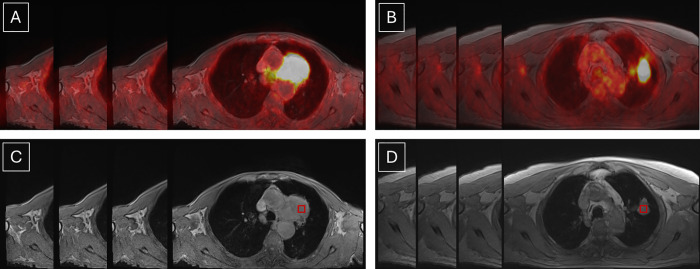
Images and selected regions of interest (ROIs) from analyzes scans of metabolically active lung tumors. ROIs were annotated as red squares. (A) PET/MRI images of adenocarcinoma (ADC), (B) PET/MRI images of squamous cell carcinoma (SCC), (C) MRI images of ADC with annotated ROI, (D) MRI images of SCC with annotated ROI.
